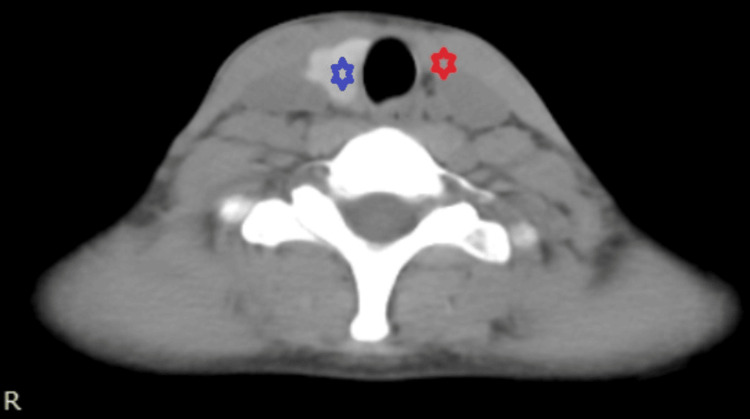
Axial Non-contrast CT Scan of the Neck Demonstrating Thyroid Hemiagenesis With the Absence of the Left Thyroid Lobe Axial non-contrast CT of the neck showing a normal right thyroid lobe, homogeneous hyperdense structure (blue star) and the absence of the left thyroid lobe with an empty left thyroid bed (red star), consistent with thyroid hemiagenesis CT: computed tomography
